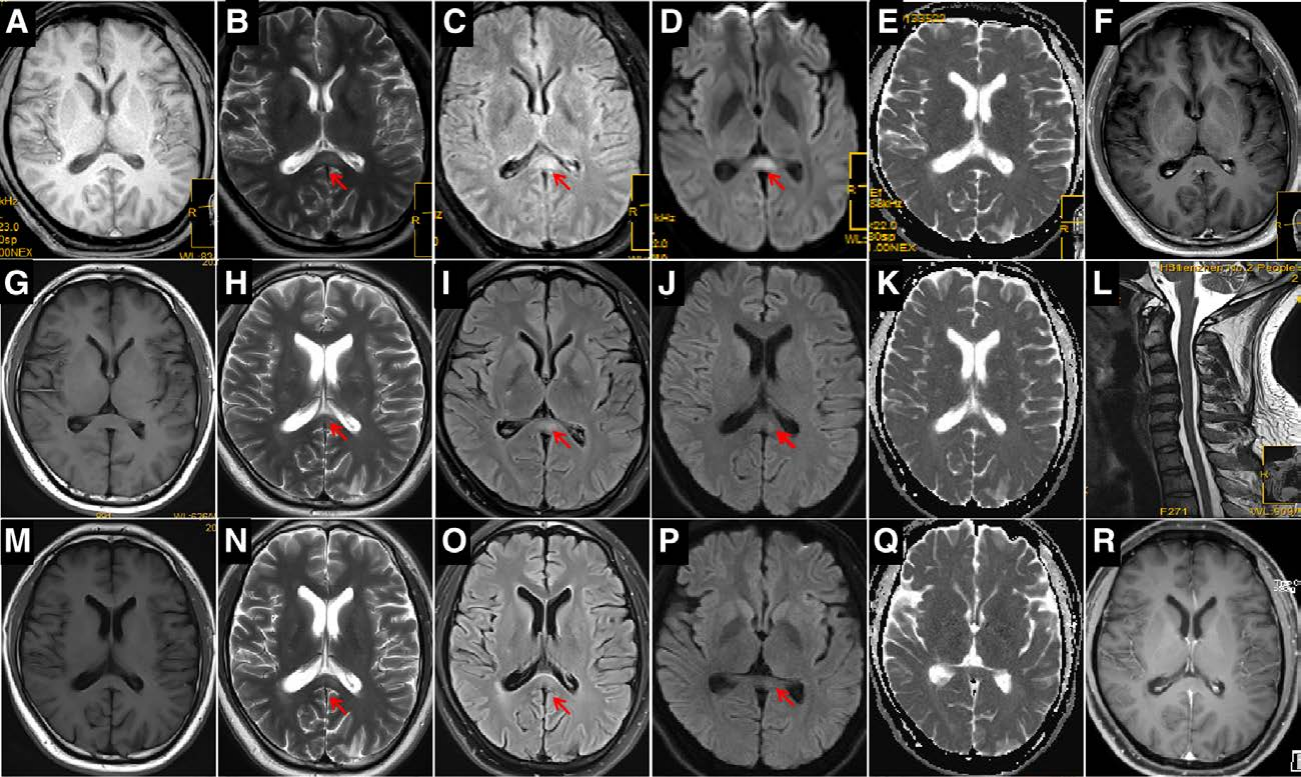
Changes in magnetic resonance imaging (MRI) findings of the patient on day 3. (a) T1-weighted imaging (WI); (b) T2-WI; (c) Fluid-attenuated inversion recovery (FLAIR); (d) Diffusion-weighted imaging (DWI); (e) Apparent diffusion coefficient (ADC); and (f) Enhanced T1-WI. (b–d), MRI displaying abnormal signals in the splenium of the corpus callosum (arrows). (g–i), MRI on day 7. (g) T1-WI; (h) T2-WI; (i) FLAIR; (j) DWI; (k) ADC; (l) Cervical spine T2-WI; (h–j), Abnormal MRI signals in the splenium of the corpus callosum display substantial improvement (arrows). (m–r), Repeat MRI at 3 weeks postdischarge. (m) T1-WI; (n) T2-WI; (o) FLAIR; (p) DWI; (q) ADC; (r) Enhanced T1-WI; (n–p), Dissipation of abnormal MRI signals in the corpus callosum (arrows). MRI = magnetic resonance imaging.
